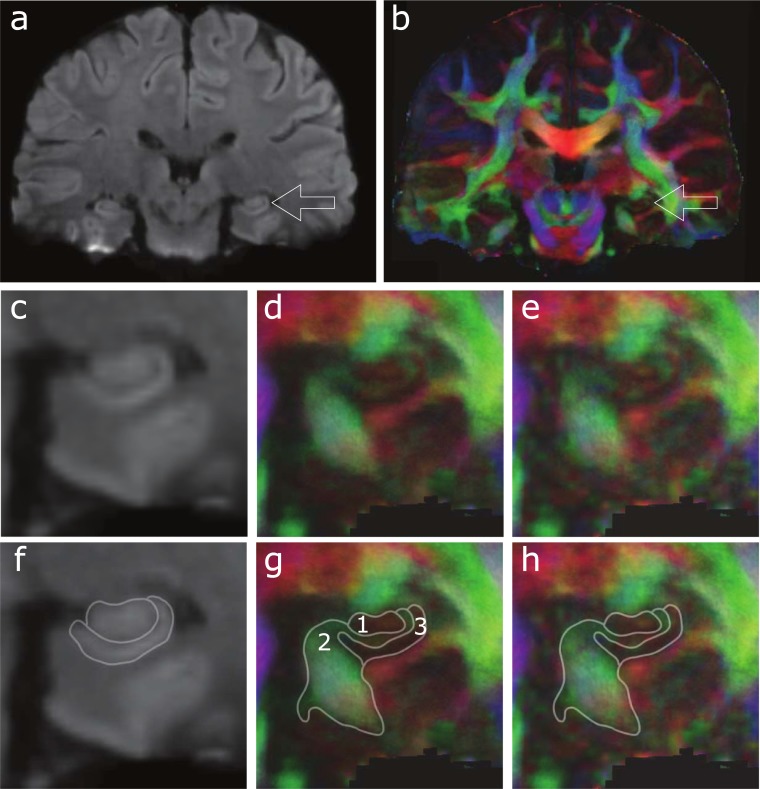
(a) and (b) show coronal-plane mean DWI and FA maps, respectively, derived from high-resolution data after DM-PCA based denoising, with arrows indicating the left hippocampus. The corresponding zoom-in images shown in (c) and (d), respectively. The FA map derived from images without DM-PCA denoising is shown in (e). The coarse hippocampal structures revealed by the mean DWI map are highlighted in (f). Anatomic structures that can be identified from color-coded FA map are shown in (g) and (h). Region 1 in (g) corresponds to the dentate gyrus; Region 2 shows fibers that connect hippocampus and entorhinal cortex to other brain areas; Region 3 contains hippocampal CA1, CA2, and CA3.
